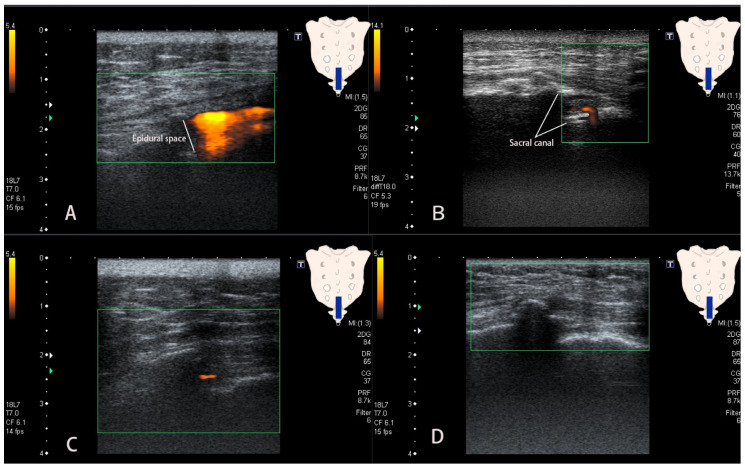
The flow signal in Power Doppler Ultrasound images. (A). Patch sign: flow filling the epidural space. (B). Earthworm sign: signal comprising a tubular flow descending through the sacral canal floor. (C). Tubular sign: signal in the sacral canal that did not fill more than half of its diameters. (D). Absent flow sign: no flow observed.
